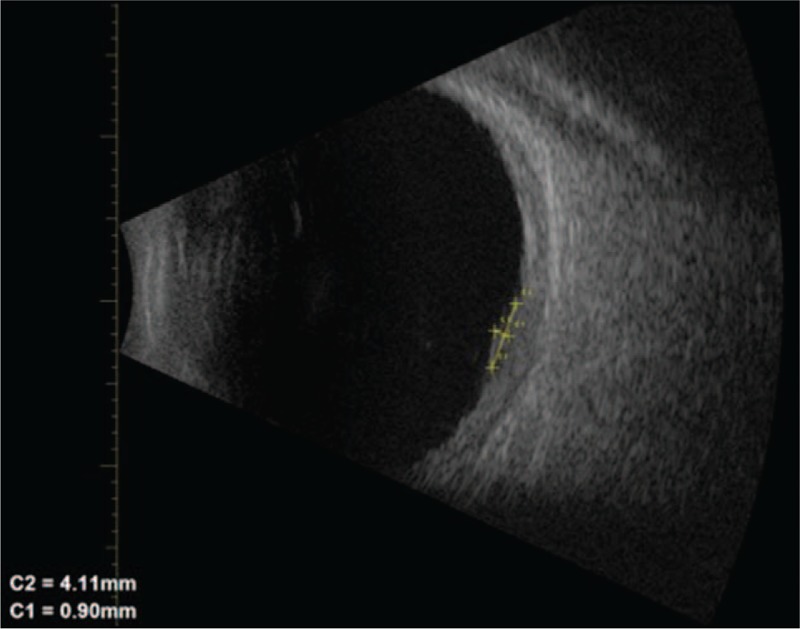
B-scan ultrasound performed when bleeding and exudation reduced significantly. Ultrasound B-scan showing a minimally elevated dome shaped, highly reflective choroidal lesion that measured 0.9 mm × 4.11 mm.
